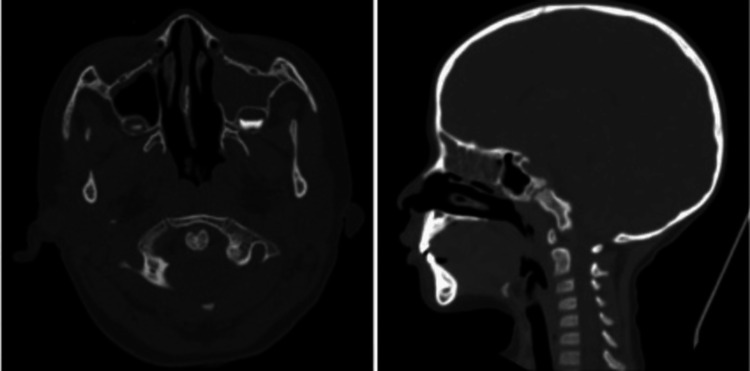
Simple CT scan after removing the foreign object showing no evidence of residual injury and air bubbles along the path of the foreign object.
